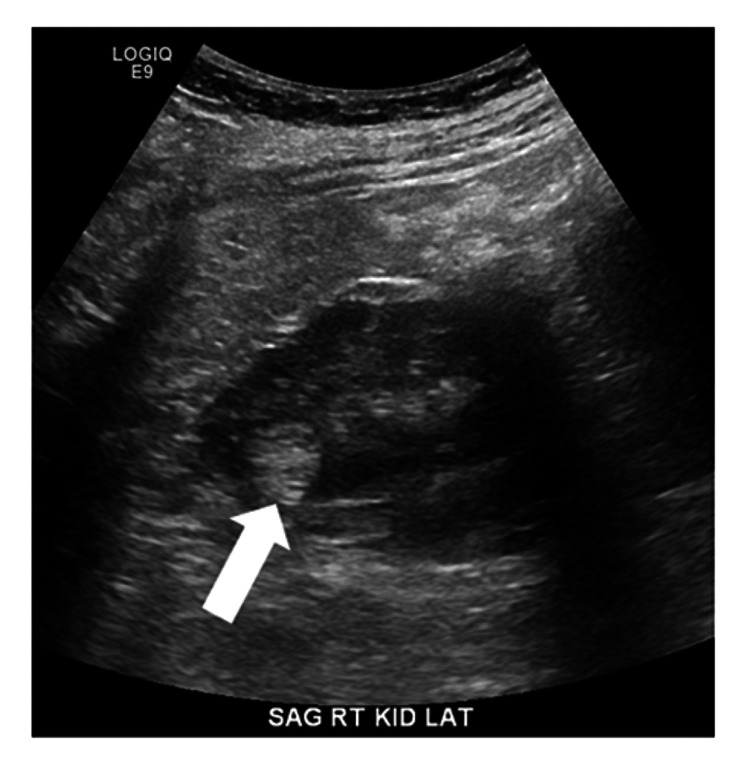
Ultrasound image of the right kidney of a woman with polycythemia rubra vera reveals an echogenic lesion interpreted as angiomyolipoma (arrow).
